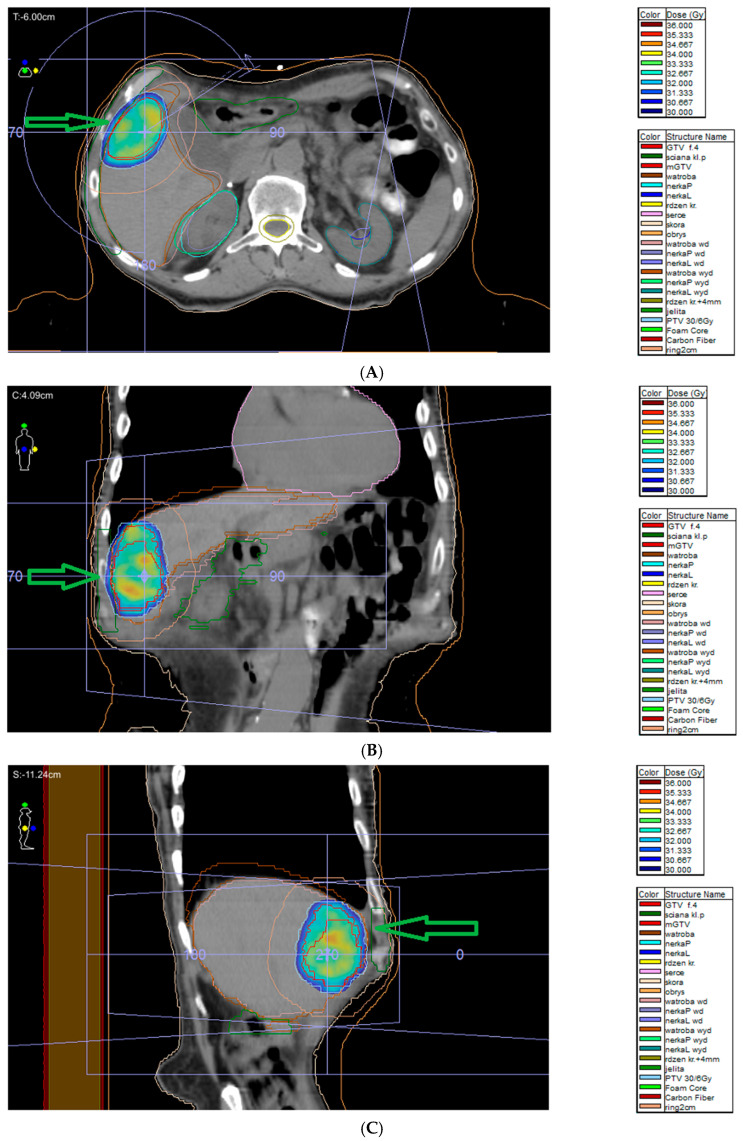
(A) Stereotactic radiotherapy for metastatic pheochromocytoma (liver metastasis) in a 73-year-old white male patient. Dose distribution shown on the CT scan; (B) stereotactic radiotherapy for metastatic pheochromocytoma (liver metastasis) in a 73-year-old white male patient. Dose distribution shown on the CT scan; and (C) stereotactic radiotherapy for metastatic pheochromocytoma (liver metastasis) in a 73-year-old white male patient. Dose distribution shown on the CT scan.
